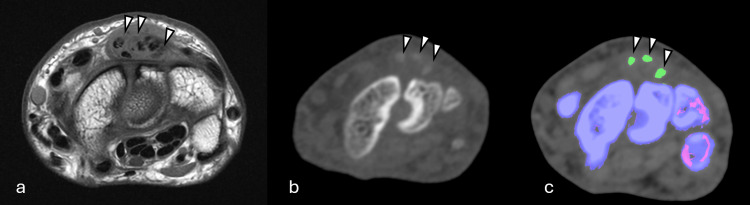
MRI, CT and Dual-energy CT images at the same level of the wrist Gouty tophi (arrowheads) appeared as low to intermediate signal in T1-weighted (T1W) MRI (a) and as mildly hyperdense foci in CT (b). They were easily picked up in dual-energy CT (c) MRI: magnetic resonance imaging; CT: computed tomography
